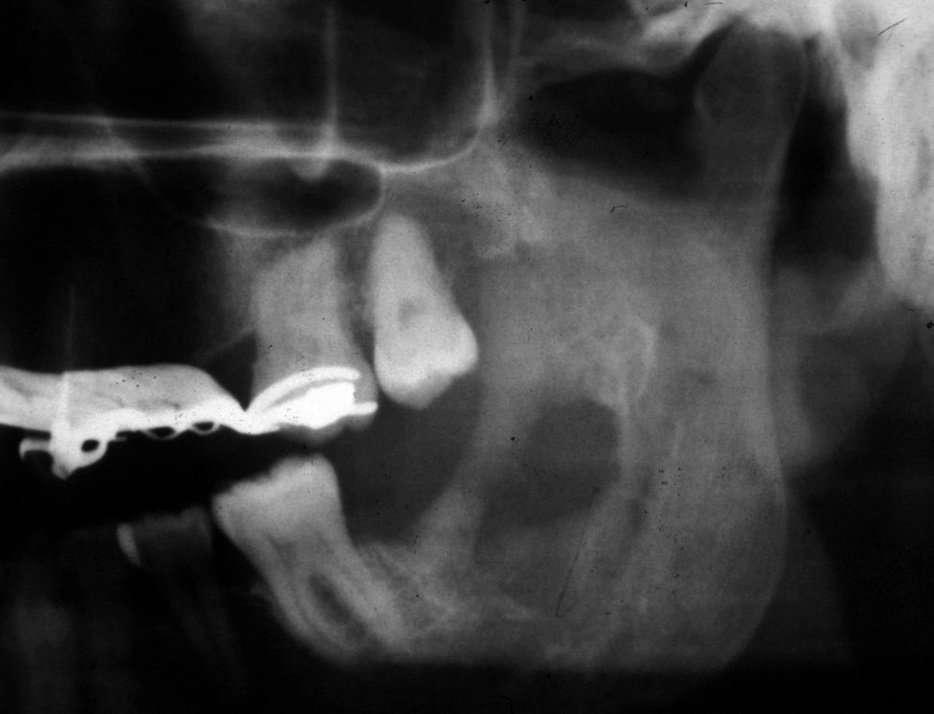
Case report 1. Panoramic X-ray at 4 months after surgical extraction of the lower left second and third molars.
